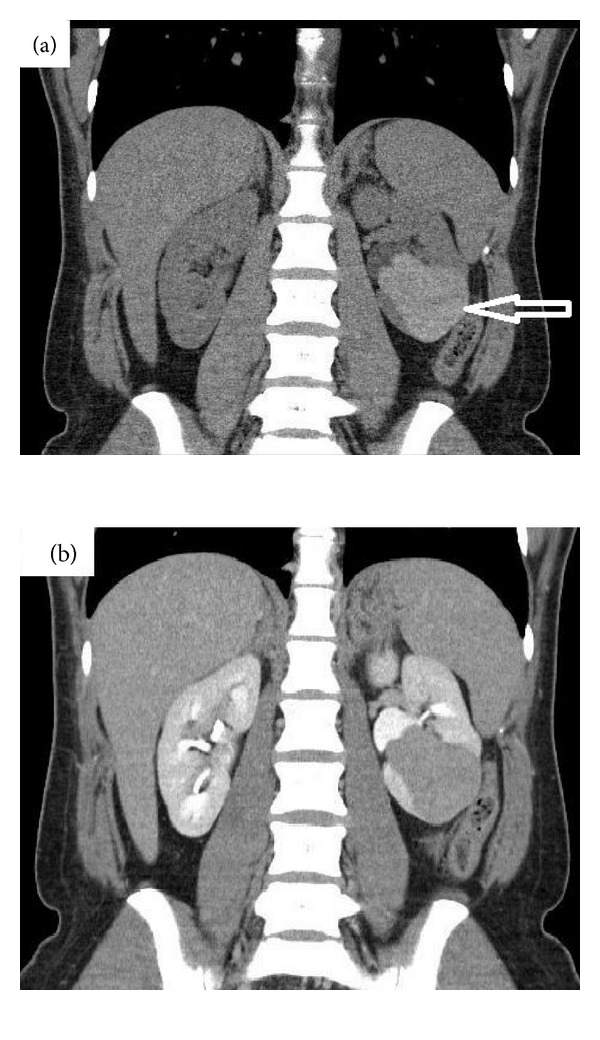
(a) Noncontrast coronal CT scan showing 5.7 × 4.9 × 5.8 cm mass in lower pole of left kidney (see arrow). (b) Postcontrast coronal CT scan showing no measurable enhancement.
